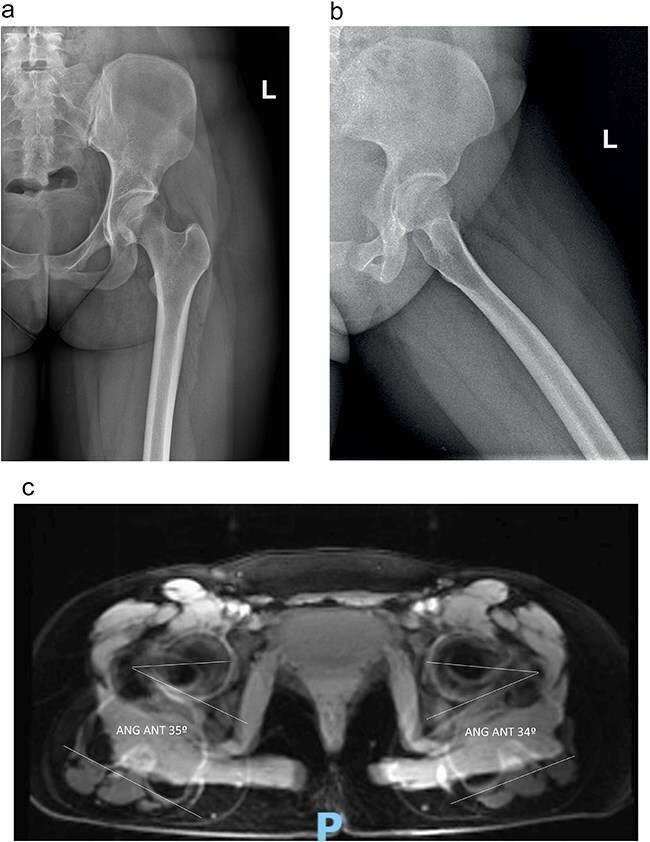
The preoperative AP (a) and lateral (b) radiographs; and the MRI scan (c) with measurement of femoral version: with high femoral version (34° left and 35° right).
